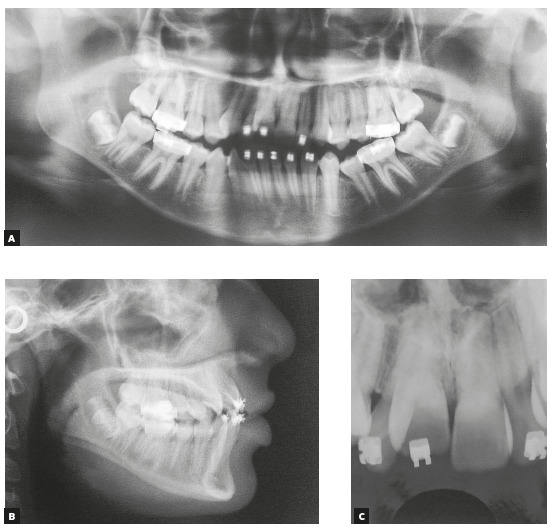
Initial panoramic, lateral, and periapical radiographs.
